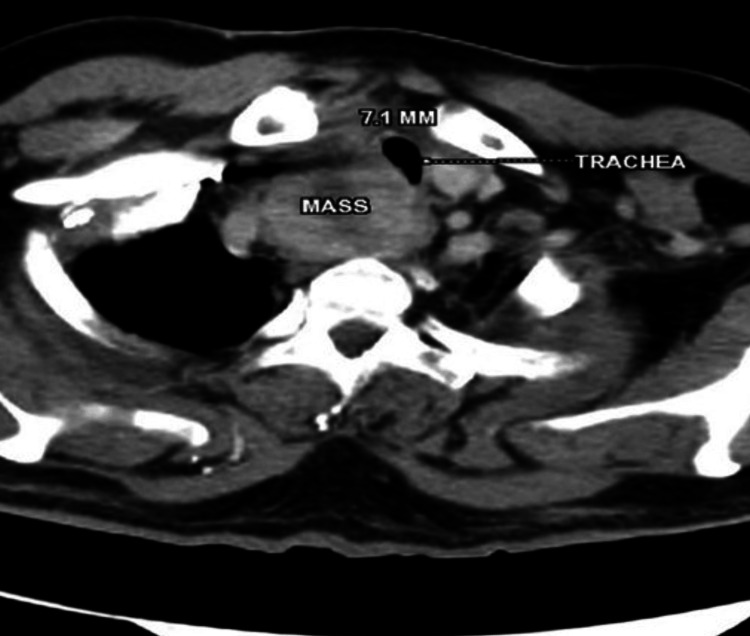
Transverse section of PET-CT at C7-D1 level. Transverse section of PET-CT showing lesion in right paraesophageal groove at C7-D1 level with compression of the trachea and narrowest lumen diameter of 7.1 mm.
